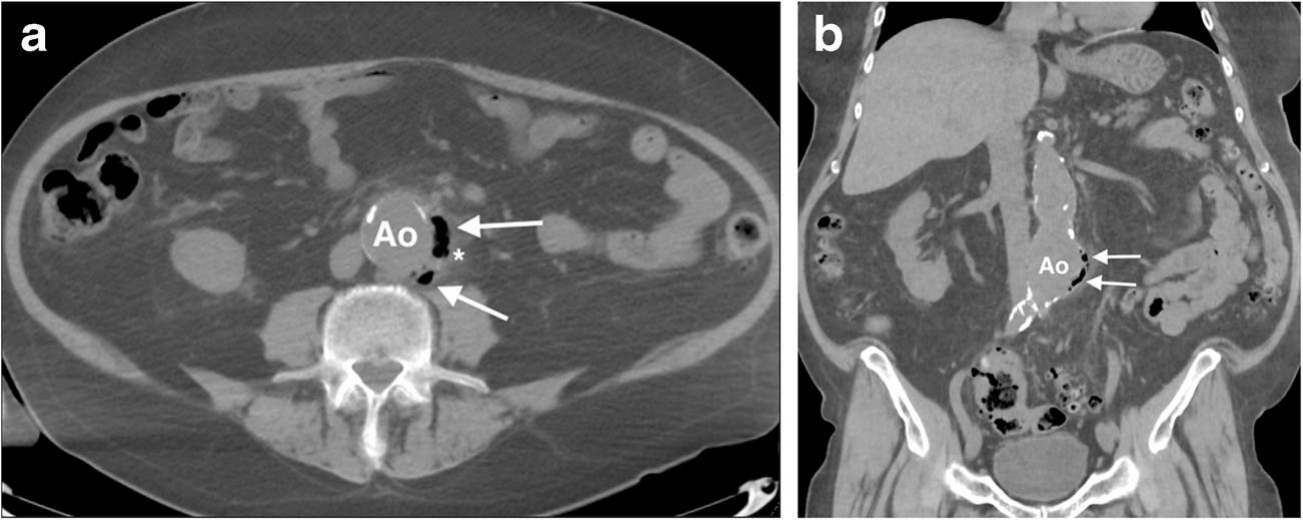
A 75-year-old man with a history of chronic kidney disease and type 2 diabetes mellitus presents with back pain and fever. (a) Axial and (b) coronal non-contrast CT scans of the abdomen demonstrates aneurysmal dilatation of the infrarenal aorta, with multiple pockets of gas within the aortic wall (*arrow*) and periaortic fat stranding (*), consistent with emphysematous aortitis. The patient underwent urgent excision of the mycotic aneurysm, with placement of an axillobifemoral bypass graft. The resected aortic wall grew *Clostridium perfringens*, a gram-positive, gas-forming anaerobic bacillus. *Ao = abdominal aorta*
